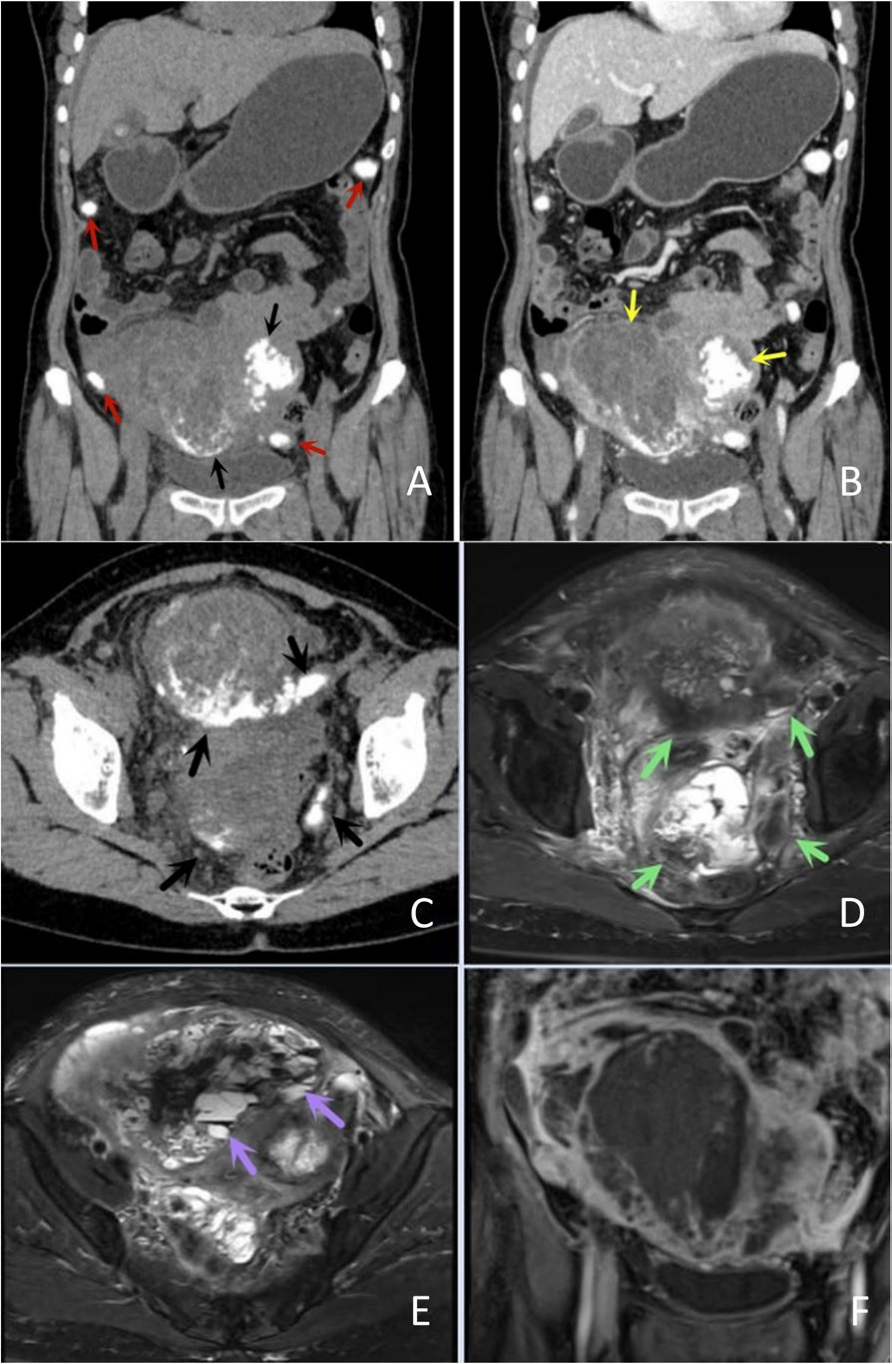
A 59-year-old woman with EOS in the uterus. A, B Coronal CT showing a large uterine mass with large, massive calcification or ossification (black arrow) and extensive cystic degeneration and necrosis (yellow arrow). Metastatic lesions with multiple calcifications in the abdominal cavity were also observed (red arrows). C, D Calcification or ossification was hypointense on MR imaging (green arrow).  F Mixed signals on T2WI, haemorrhage within the mass, and a fluid‒fluid level (purple arrow) were observed. B, F The enhanced CT and MR findings were consistent, showing less uniform and significant enhancement
